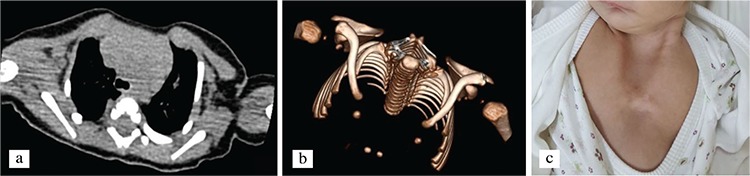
Axial section of preoperative computed tomography scan showing the partial superior sternal cleft with the complete lack of fusion of the sternal bars (a). Three-dimensional reconstruction showing the split sternum bones with the ossification center (b). Preoperative view of the anterior chest Wall (c).
